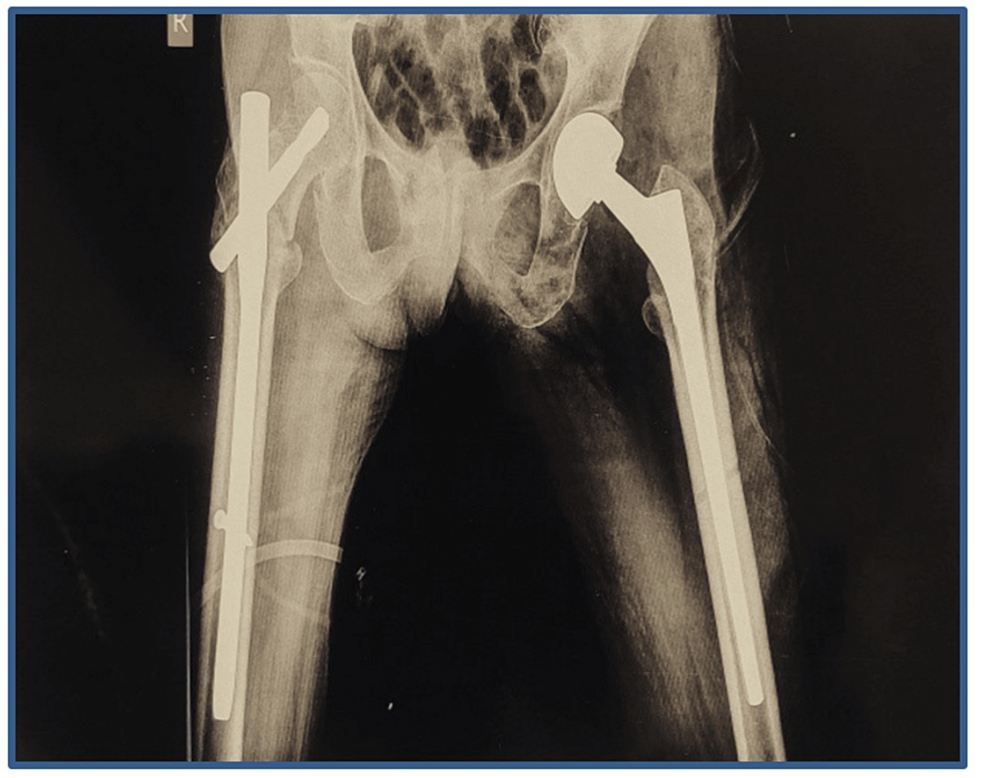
Postoperative X-ray of the pelvis with both hips anterior-posterior operated with bipolar hemiarthroplasty
